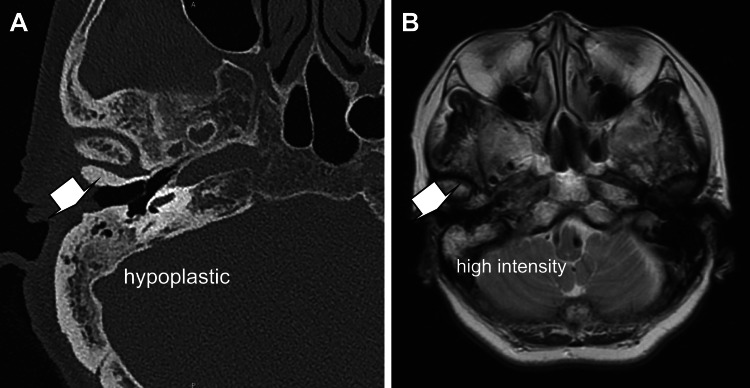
Computed tomography and magnetic resonance imaging of the head A. The right mastoid apicitis was hypoplastic, and chronic otitis media or mastoid apicitis was suspected. B. High-intensity findings in the right mastoid process on T2-weighted imaging suggest inflammation within the mastoid endoplasmic reticulum.
